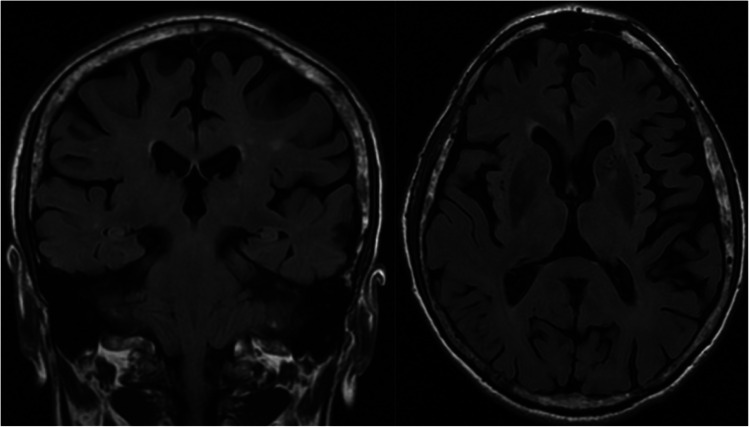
MRI analysis of PD patient. Three-dimensional T1-weighted images showing widespread cortical atrophy in a PD patient carrying the TMEM175 p.P14L mutation (coronal slice, left; axial slice, right)
